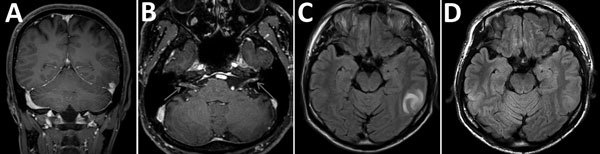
Brain magnetic resonance imaging findings in a 36-year-old immunocompetent man before (A, B, C) and after (D) treatment for cerebral syphilitic gumma, Saitama, Japan. A) Gadolinium-enhanced T1-weighted coronal image shows an enhanced nodular lesion in the left temporal lobe. B) Axial gadolinium-enhanced T1-weighted image shows enhancement within the cisternal segment of both the vestibulocochlear nerve complex and the facial nerve. C) Axial fluid-attenuated inversion recovery images show a hyperintense lesion-like mass in the left temporal lobe. D) Axial fluid-attenuated inversion recovery image shows complete resolution after discontinuation of treatment.
